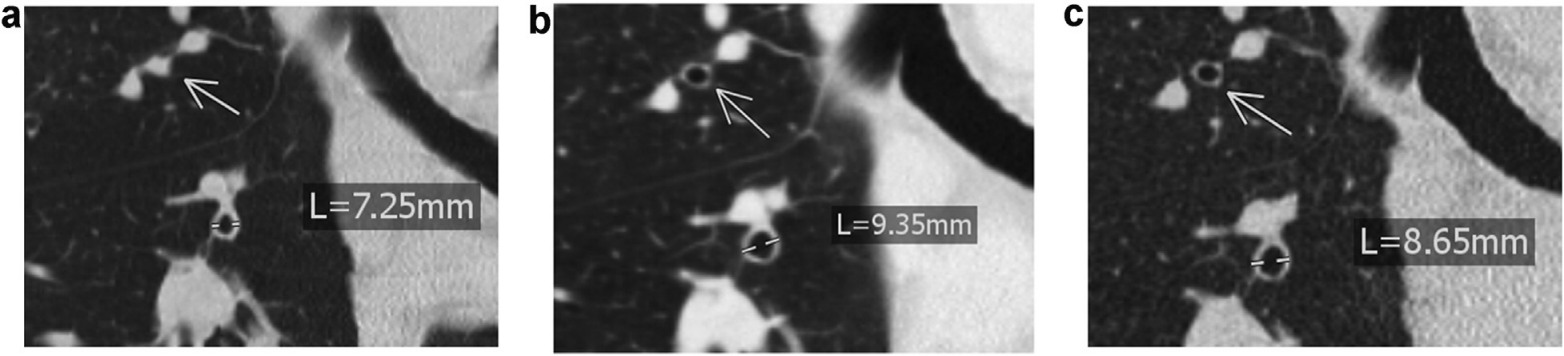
Comparison of CT findings in 2017 (a), January 2021 (b), and September 2021 (c) shows a decrease of mucous plugging (arrow) and bronchial wall thickening as well as an increase in bronchial caliber
